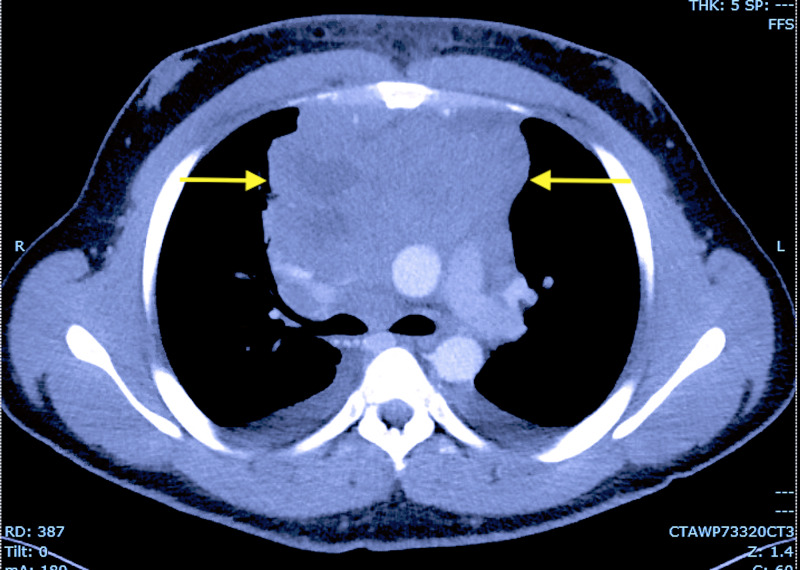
Computed tomography of the chest showing a large anterior mediastinal mass (yellow arrows) with compression of the superior vena cava.
